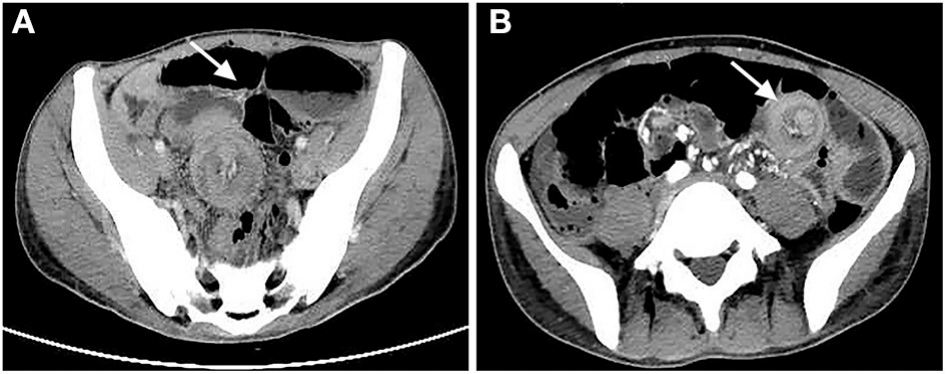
(A) Abdominal CT scan first noticed intestinal obstruction with sign of air-fluid level instead of intussusception. (B) Transverse section of CT scan indicated intussusception with sign of “target-like” or “concentric double ring” (both are indicated by white arrow above).
